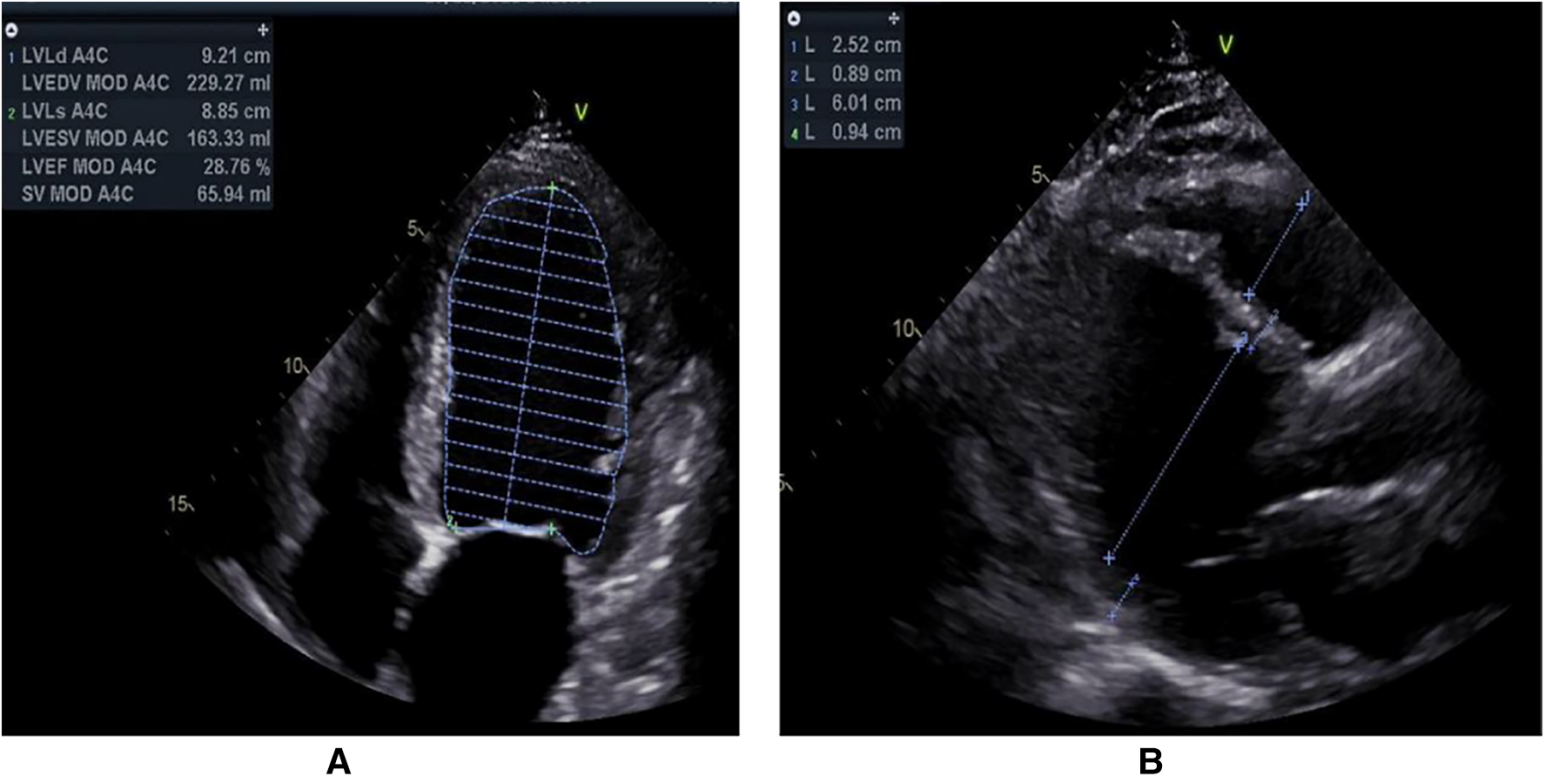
Transthoracic echocardiography was routinely examined from left parasternal long-axis view (A) and apical four-chamber view (B).
